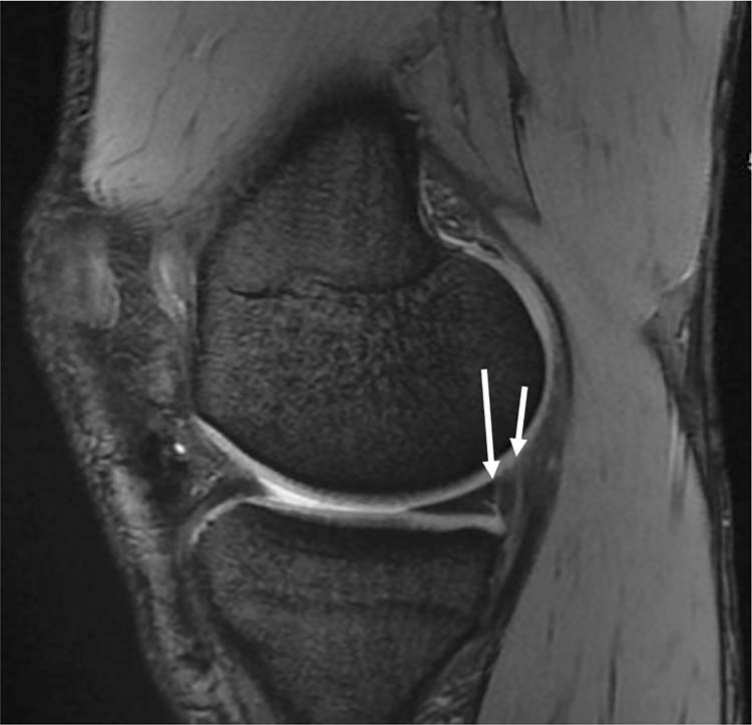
Sagittal magnetic resonance imaging of the knee. Gradient echo sequence image of the medial compartment of the knee demonstrating a suspicious double ramp lesion. The long arrow indicates the first ramp lesion, and the short arrow indicates the second ramp lesion
